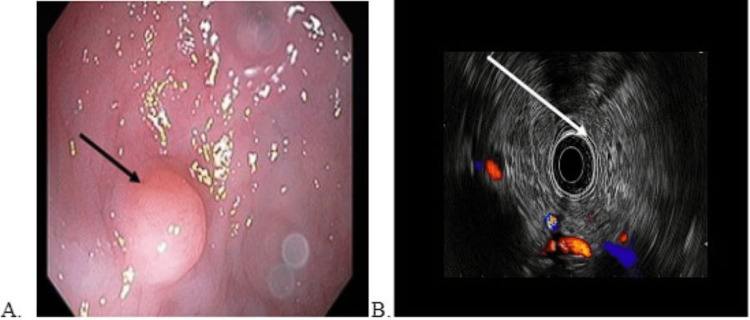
A) Colonoscopic visualization of the mass in the rectum; B) Endoscopic image of the lesion during EMR via EUS EMR: endoscopic mucosal resection; EUS: endoscopic ultrasound
